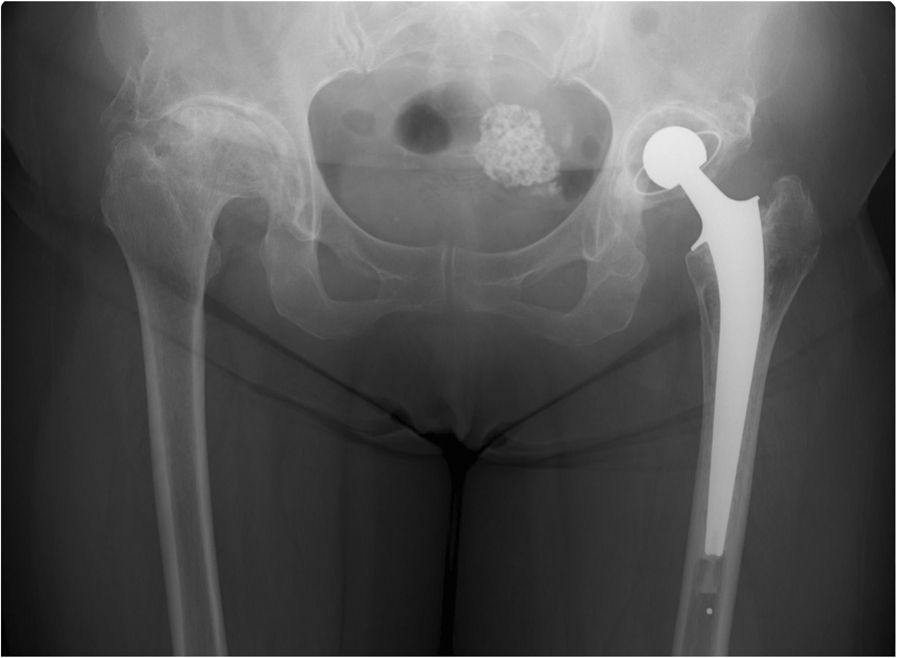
Plain radiography of the bilateral hip joints at 3 years and 6 months after surgery. Bone fusion of the periacetabular osteotomy pseudoarthrosis region is complete at 3 years and 6 months after the surgery. No loosening of the acetabular cup or stem was observed at 4.5 years after surgery
